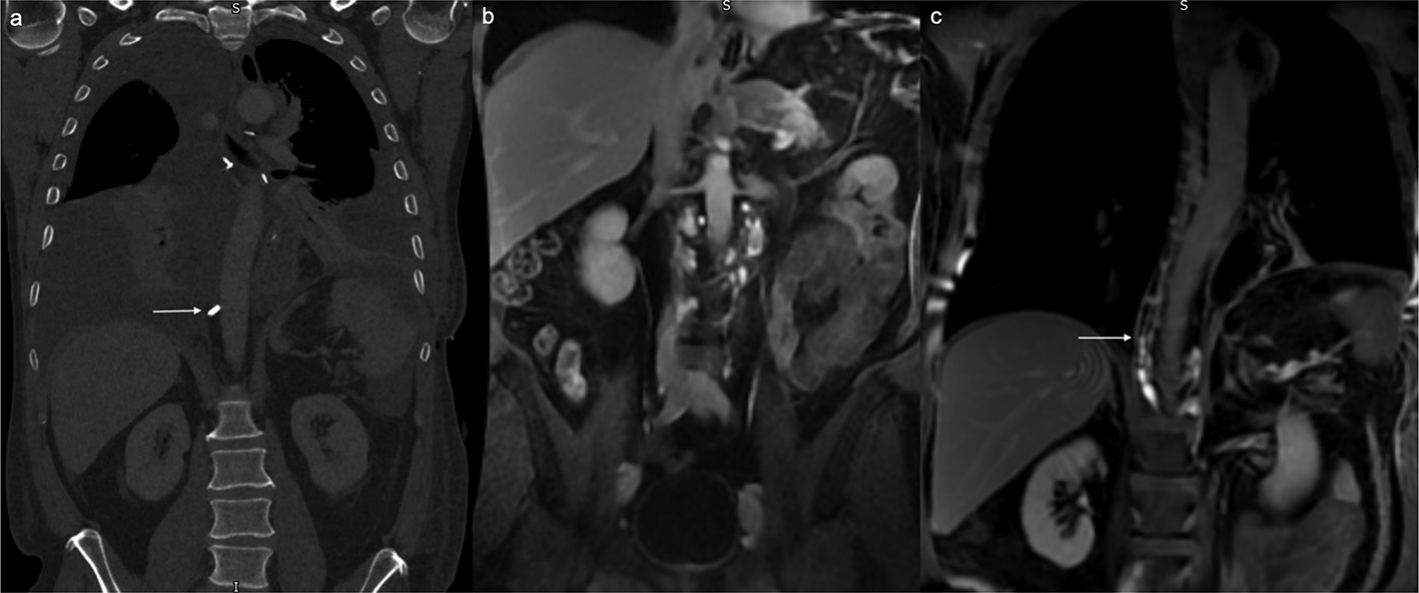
Patient with bilateral pleural effusions post-oesophagectomy on coronal CT with thoracic duct ligation clip (Figure 4a, white arrow). There was progressive enhancement of the abdominal lymphatics, cisterna chyli and inferior thoracic duct up to the level of T11-12 (Figure 4b–c, white arrow), which corresponds to the level of thoracic duct ligation clip on the coronal CT. Contrast did not extend cranially on delayed imaging, in keeping with a ligated thoracic duct.
